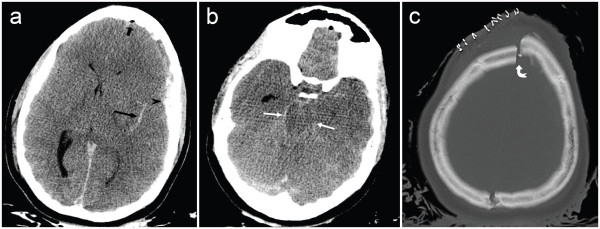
Computed tomography images of the patient’s head. (a, b) Unenhanced axial computed tomography images demonstrate subdural (arrowhead) and subarachnoid hemorrhage (long black arrow), pneumocephalus (short black arrow), midline shift to the right, and transtentorial herniation (white arrows). (c) Numerous skull fractures were present, including a fracture through the sagittal suture with diastasis through the midline skull (curved white arrow).
